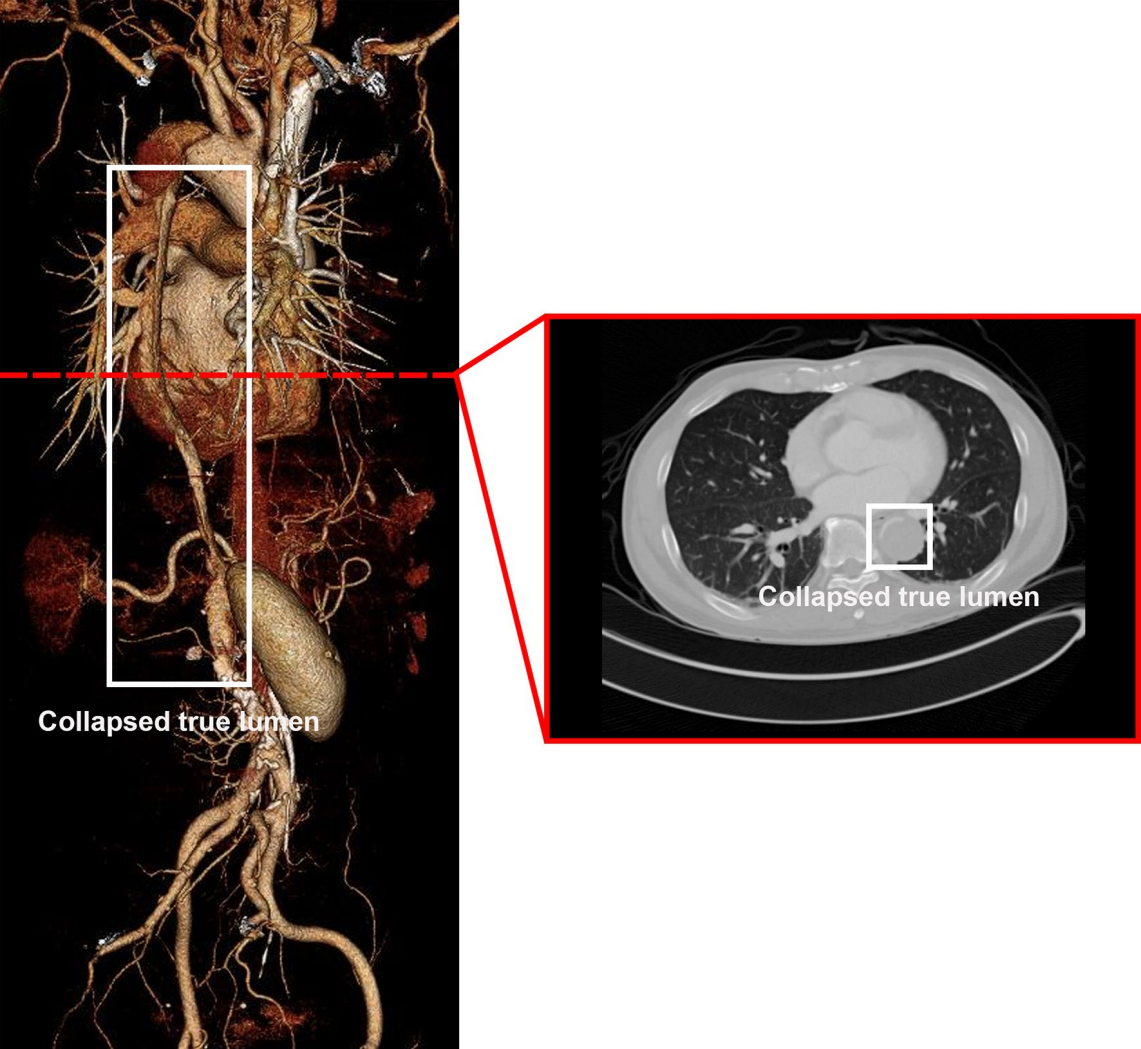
3D volume rendering and axial view of computed tomography in an AD patient with lower extremities malperfusion due to true lumen collapse. The image was reconstructed using TeraRecon (https://www.terarecon.com).
